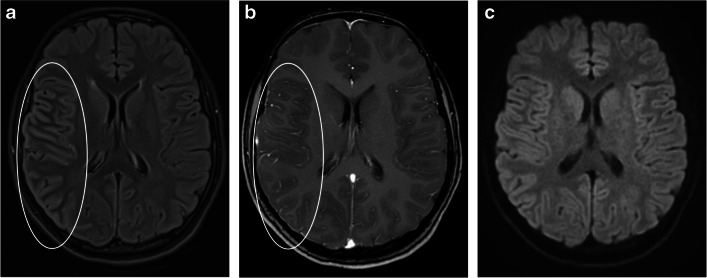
Fluid-attenuated inversion recovery (FLAIR) hyperintense lesions in anti-myelin oligodendrocyte glycoprotein encephalitis with seizures (FLAMES): magnetic resonance images of the brain, obtained from a 14-year-old girl, presented with right ocular symptoms. a Axial fat-suppressed FLAIR image shows an increased signal along the right peri-Sylvian and parietal cortex with sulcal effacement (*ellipse*). b Axial contrast-enhanced T1-weighted image shows associated leptomeningeal enhancement (*ellipse*). c On axial diffusion-weighted image (*b*=1,000), no diffusion restriction is seen. Images courtesy of Dr. Elena Zharkov, Pediatric Radiology Unit, Shaare Zedek Medical Center, Jerusalem, Israel
